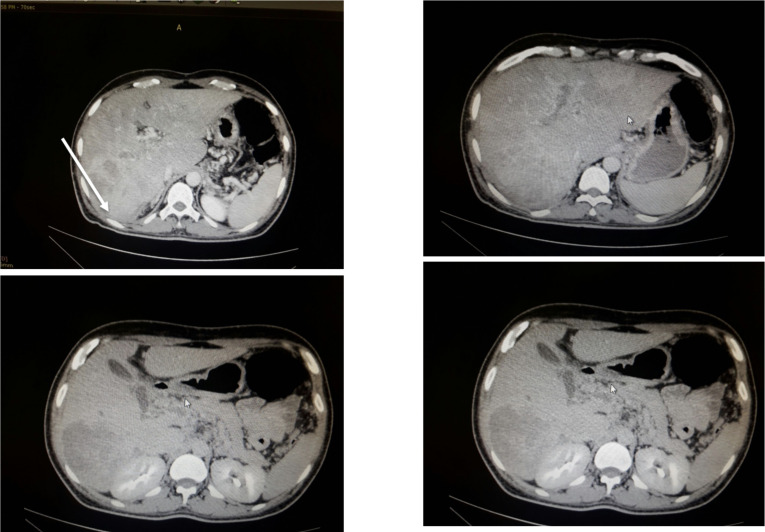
Tri-phasic abdominal CT scan 6 months after the initial abscess treatment (on the second admission to the Hospital)
